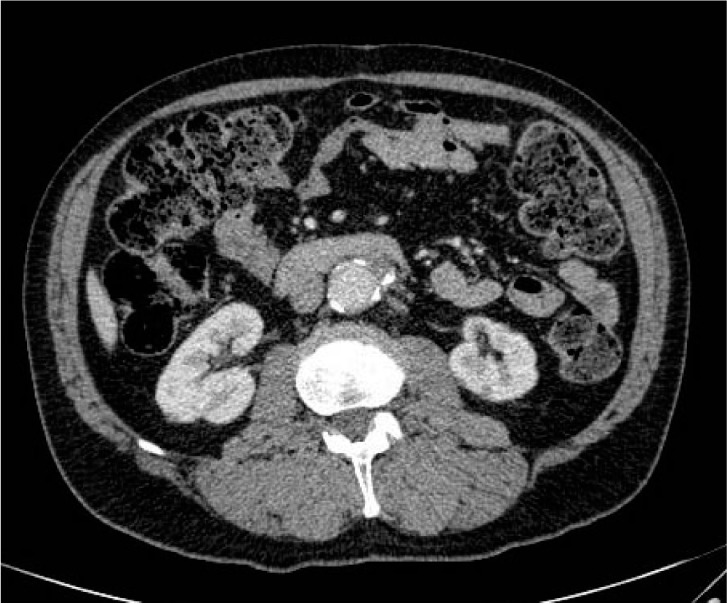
Computed tomography of tumour at 17 months after surgery: The upper aspect of the tube graft with collapsed aneurismal sac surrounding it can be seen; the left lower pole renal lesion is no longer demonstrable.
